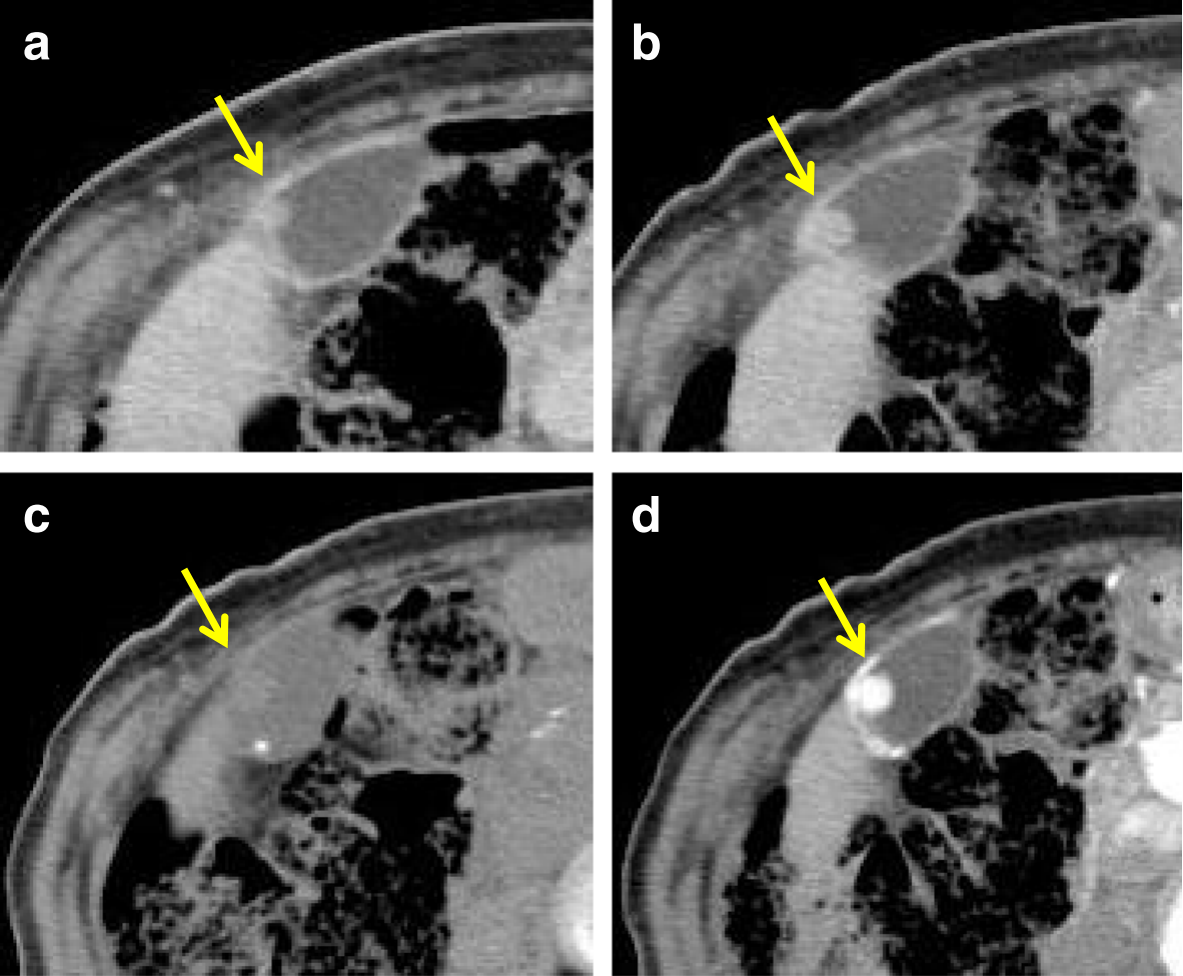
A computed tomography scan shows tumor growth from 4 mm to 12 mm over a span of 2 years (a 2 years prior, b present). A contrast-enhanced computed tomography scan shows high intensity of the tumor during the arterial phase (d
